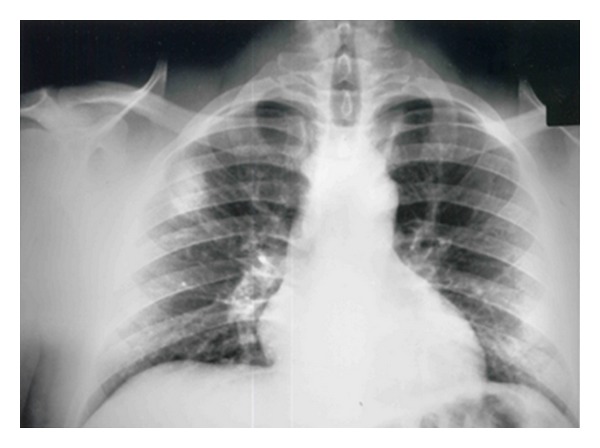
Chest X-ray revealing a rounded opacity in the right upper lobe.
